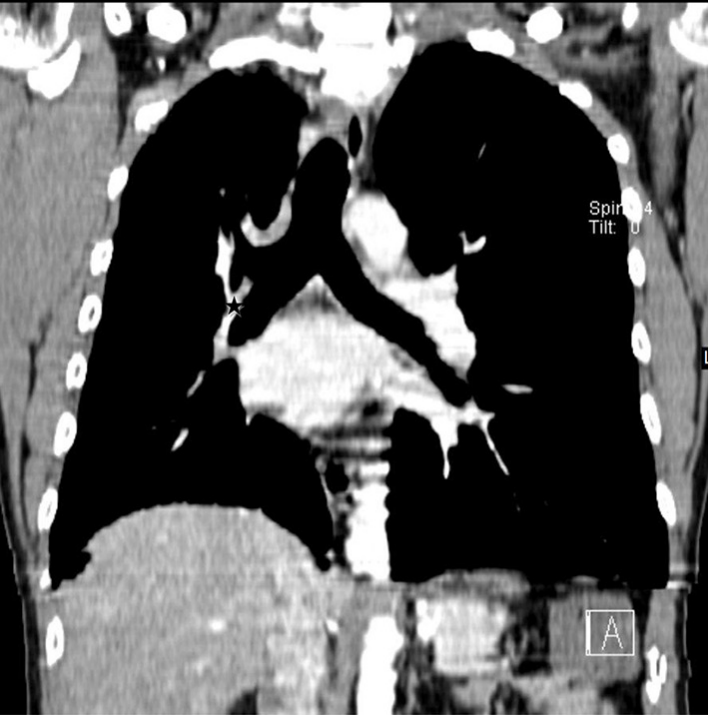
Coronal reformatted images of contrast-enhanced CT chest reveals absence of the mediastinal portion of the right PA (
